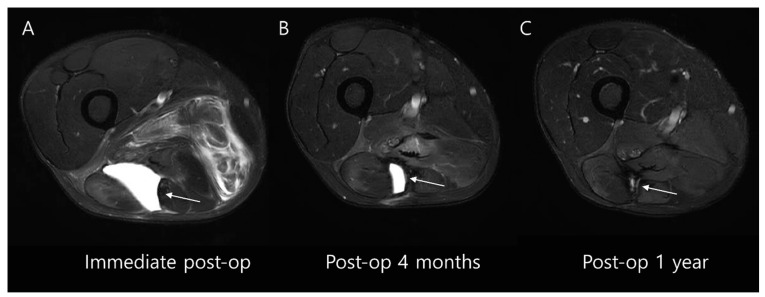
Postoperative seroma following wide excision of atypical lipomatous tumor in the thigh. (A) Immediate postoperative axial T2-weighted fat-suppressed MR image shows a well-defined, homogeneous fluid collection at the surgical site (arrow). (B) Axial T2-weighted fat-suppressed MR image taken 4 months after tumor resection demonstrates partial reduction in the size of the fluid collection (arrow). (C) One year post-surgery, axial T2-weighted fat-suppressed MR image shows near-complete resolution of fluid collection within the surgical site (arrow).
